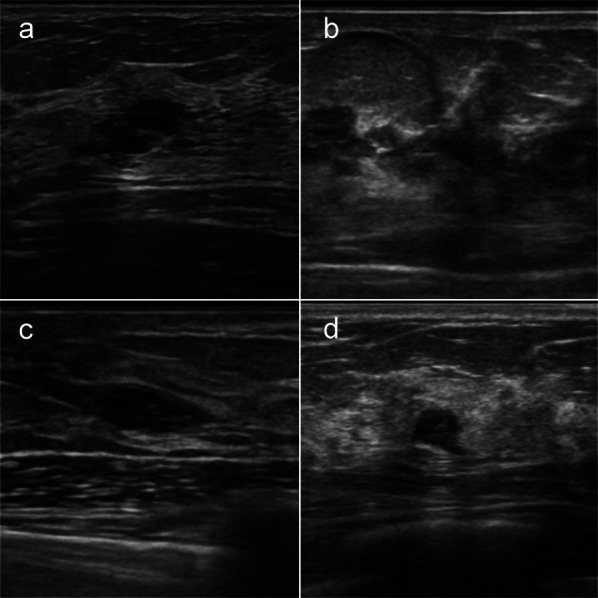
Ultrasonographic image. a Dilated duct collection on Case 1. b Distortion on Case 2. c A circumscribed lobulated hypoechoic mass on Case 3. d A microlobulated irregular hypoechoic mass on Case 4
